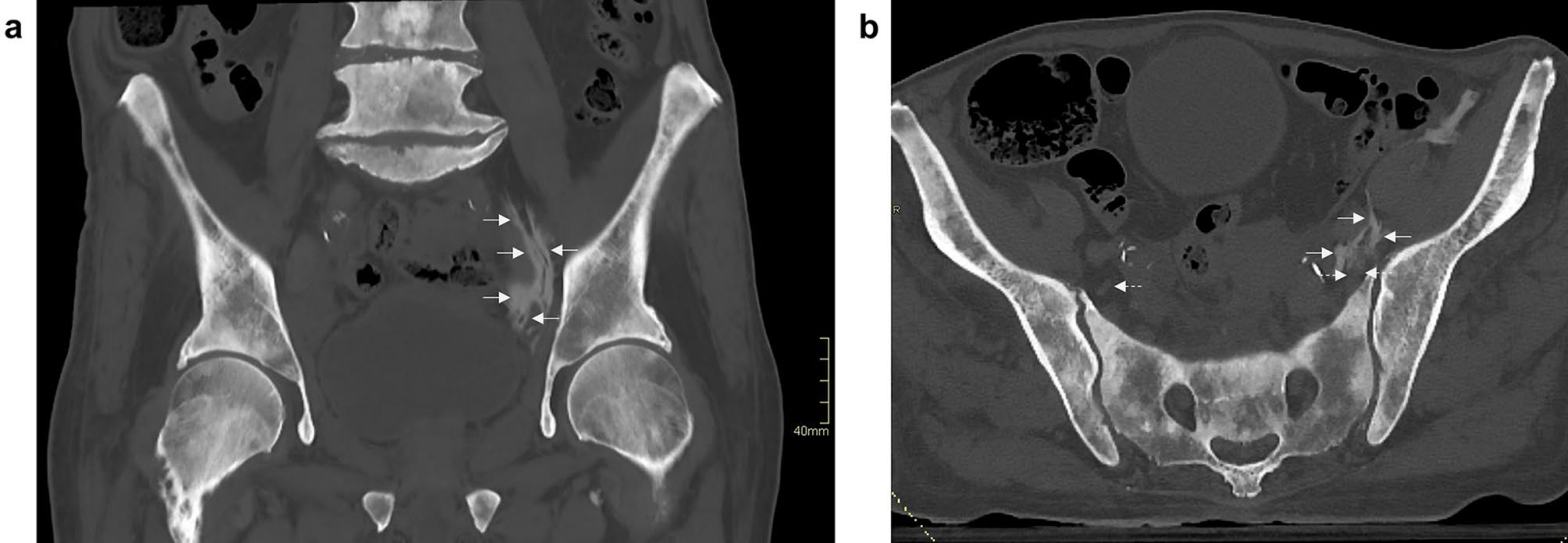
Coronal (a) and axial (b) CT imagery of the S-FICB case wherein the contrast mixture reached the ON (left side). The injectate was found to involve the ON in the retroperitoneal compartment at the level of the first sacral vertebra, which was also the maximum height that was reached. Dashed arrows point to the ON, while solid arrows point to contrast.
